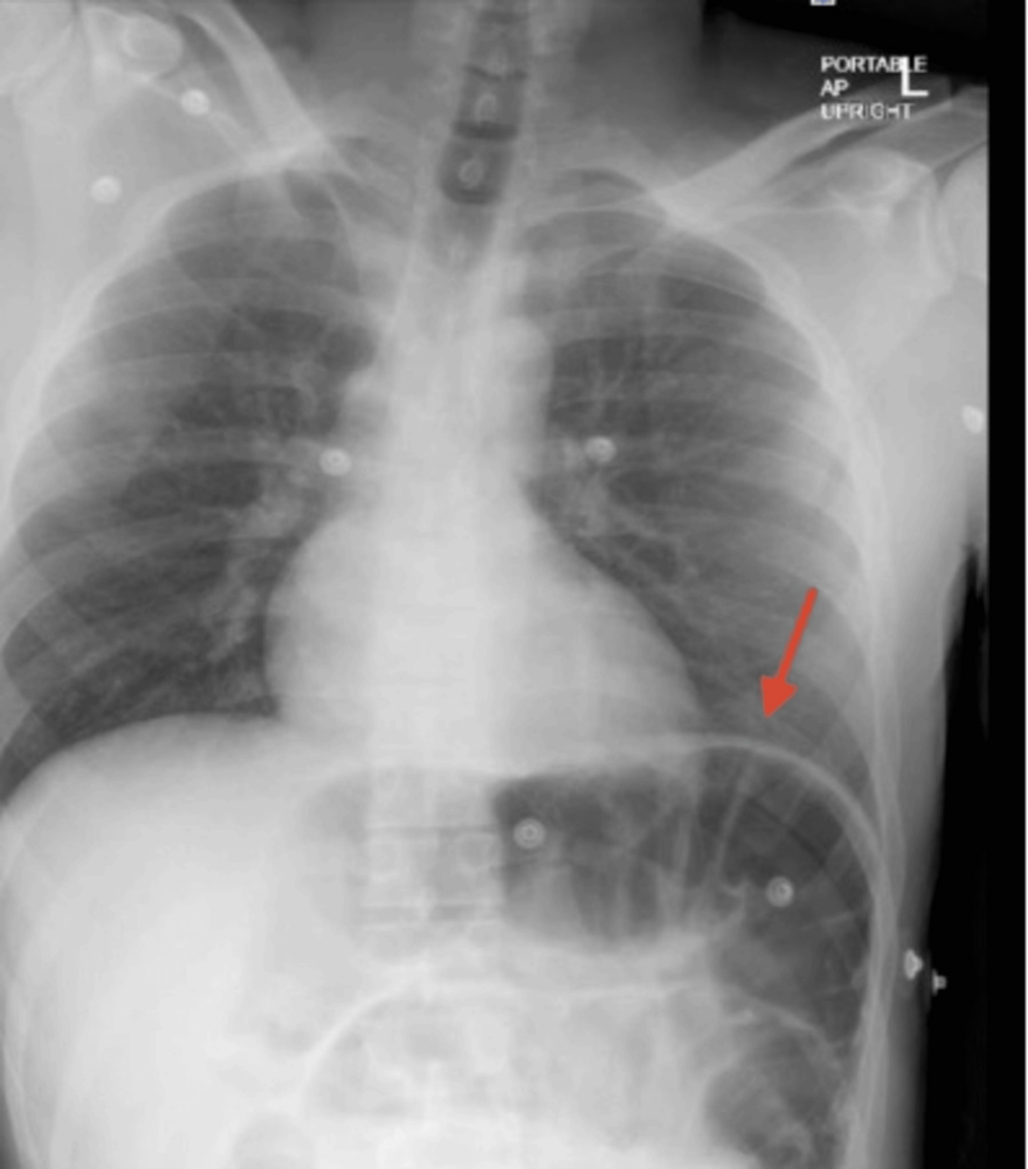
Chest X-ray showing dilation of the bowel The chest X-ray reveals marked gaseous bowel dilation in the left upper quadrant (red arrow), raising concern for an underlying obstruction.
